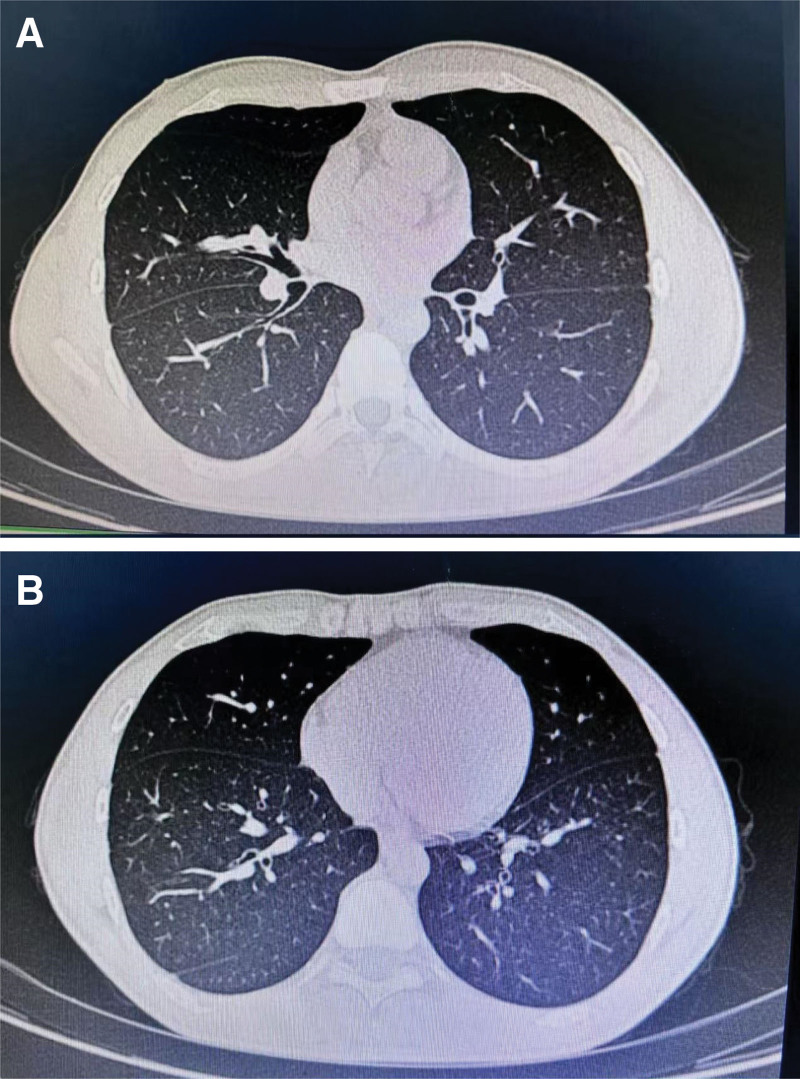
(A1, A2) Preoperative chest CT scans
